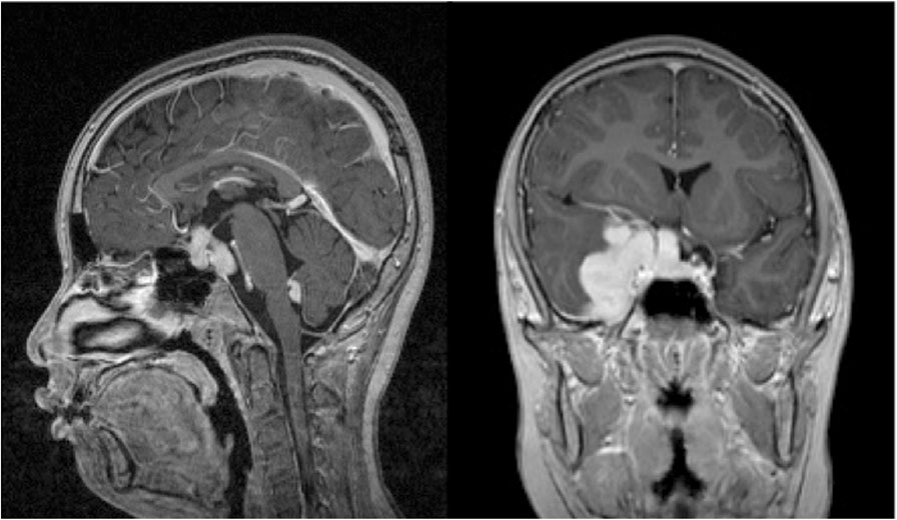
Sagittal T1-weighted MRI with gadolinium (left) demonstrating a small enhanced mass of the fourth ventricle and an homogeneous enhanced mass in the pituitary and infundibulum. Further, coronal T1-weighted MRI with gadolinium (right) revealed extensive involvement of the right cavernous sinus and the presence of dural enhancement resembling a dural tail sign in the right middle cranial fossa
